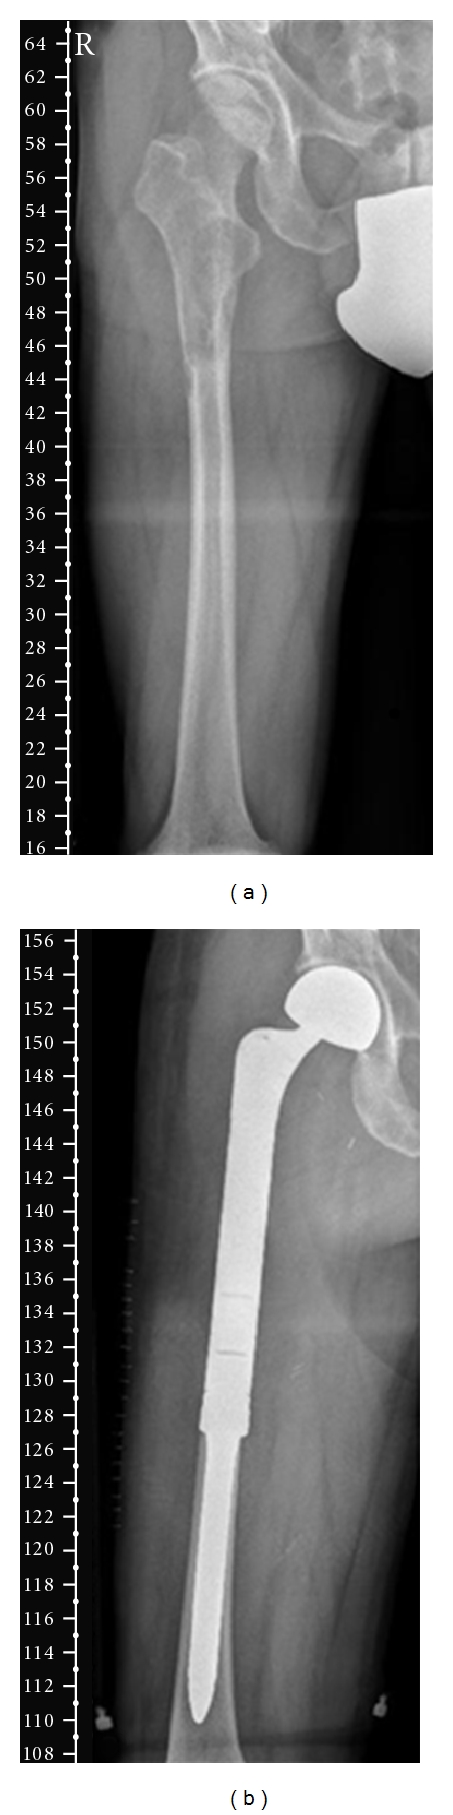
Radiographs of the patient's right femur: (a) preoperative, an expanding lytic lesion (10 cm in max. diameter) located in the right proximal femur with extension into the proximal shaft, (b) two months after limb salvage surgery followed by endoprosthetic replacement.
